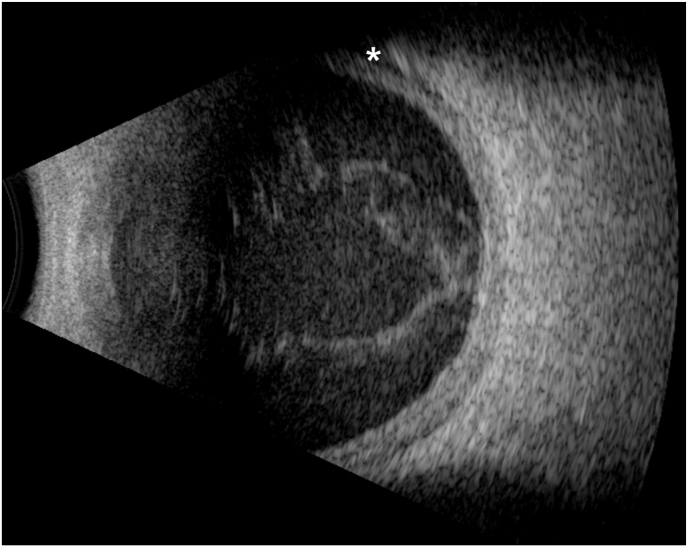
B-scan ultrasonography at first episode B-scan ultrasonography of an 83-year-old male with suspected post-intravitreal injection-associated endophthalmitis demonstrating vitreous opacities and membrane formation (arrows) with a shallow choroidal detachment (asterisk).
